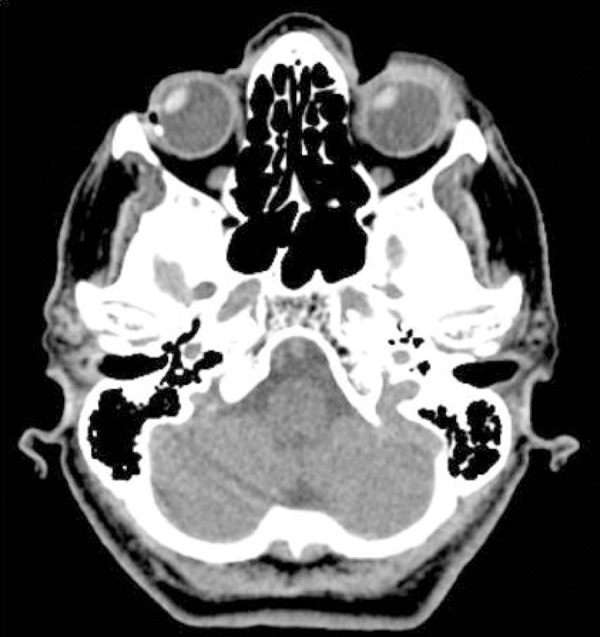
Non-contrast computed tomography (CT) scan showing conjugate eye
